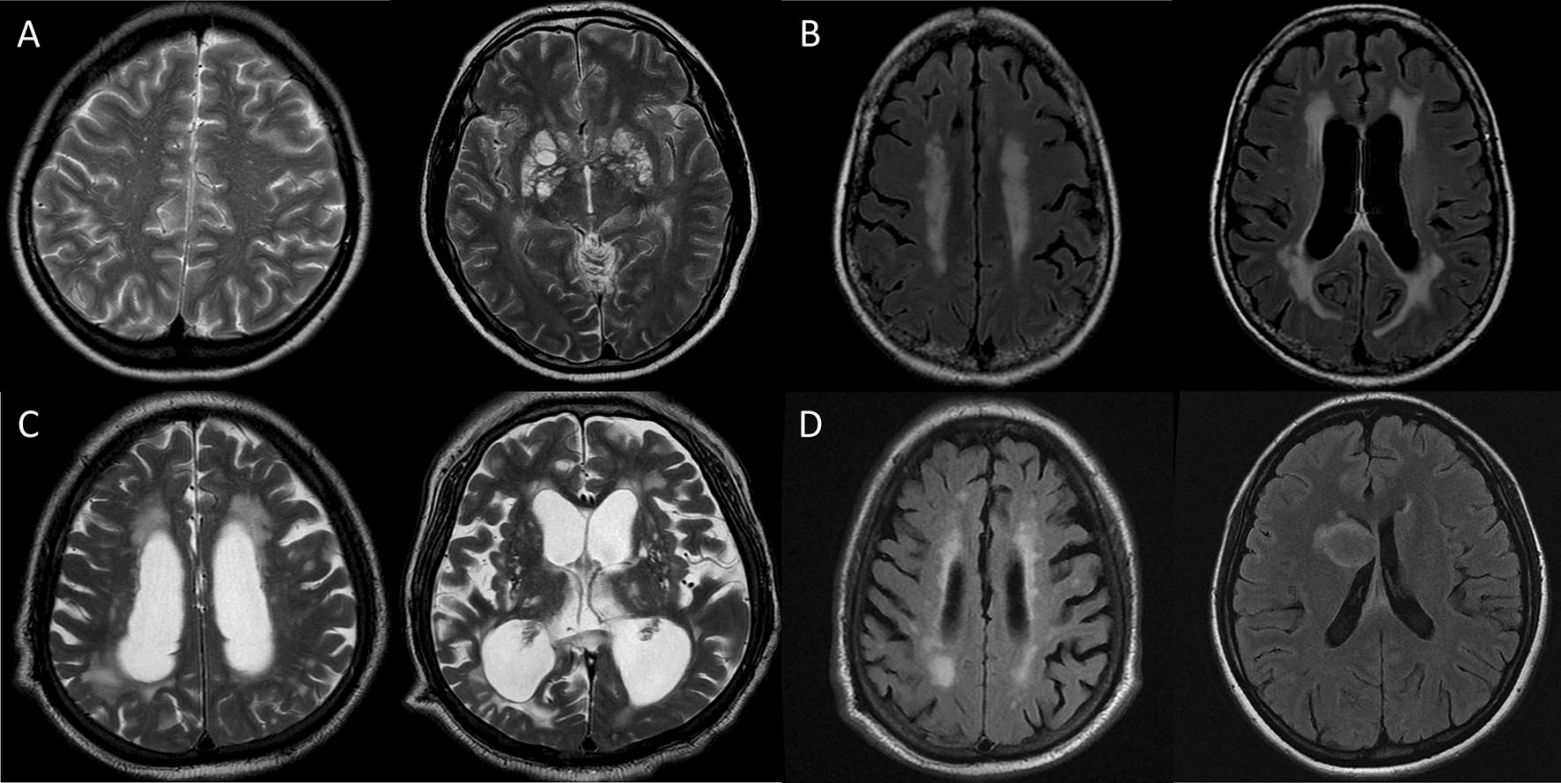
Examples of the brain MRI findings associated with cryptococcus meningoencephalitis. Panel (A), enlarged periventricular space (ePVS) in centrum semiovale (> 40 EPVS, score = 4, left panel) and basal ganglia (> 40 EPVS, score = 4, right panel); Panel (B), periventricular extension of the T2 high-signal intensity (HSI) on T2 fluid attenuated inversion recovery (FLAIR) images; Panel (C), hydrocephalus (Evans ratio = 0.42); and Panel (D), development of multiple cryptococcomas in right parietal cortex (left panel) and right caudate nucleus (right panel, T2 FLAIR images).
